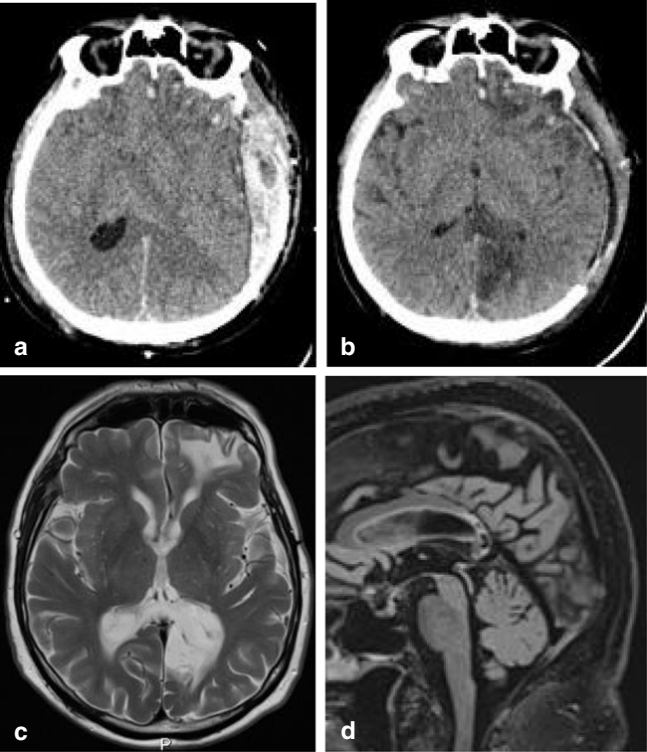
Sixty-six-year-old man. Axial CT with leftsided subdural hematoma and diffuse brain swelling without splenial hypodensity on day of trauma (a); 3 days later, infarction is seen in the left side of the splenium and in the left occipital lobe (b). At follow-up, 7 years later, the patient has no residual cognitive disturbance. Axial T2 (c) and sagittal 3D FLAIR (d) show extreme tissue loss in the same regions
